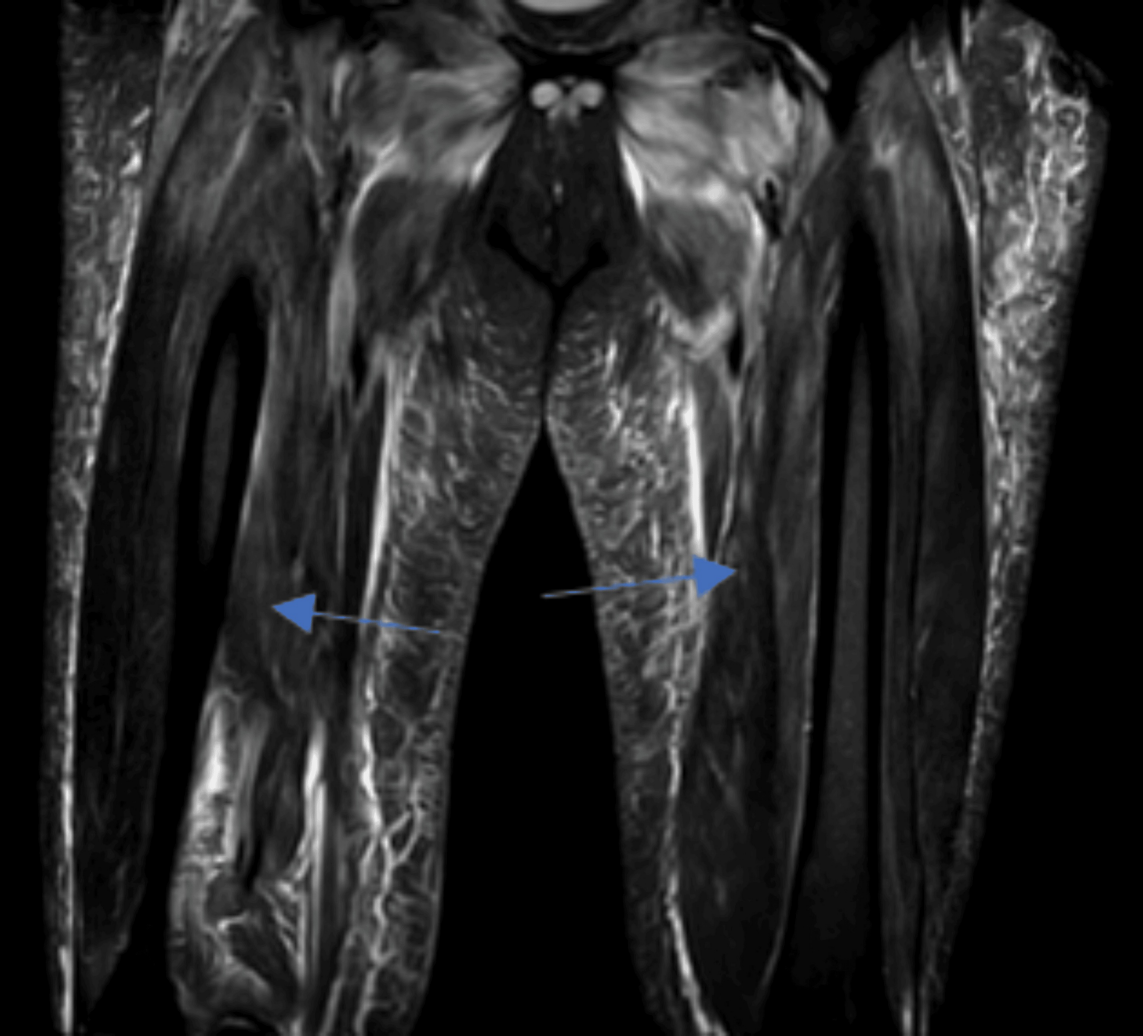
T2-weighted MRI showing the presence of edema in bilateral thighs
